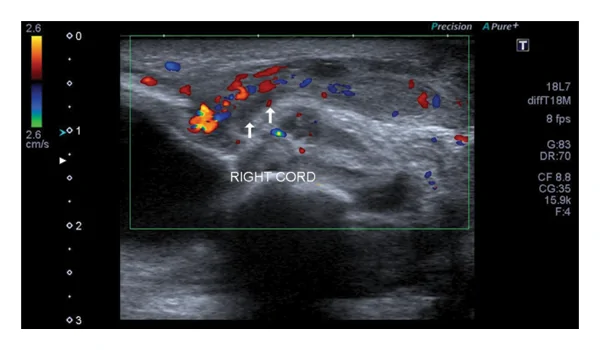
Postoperative color Doppler ultrasound.
